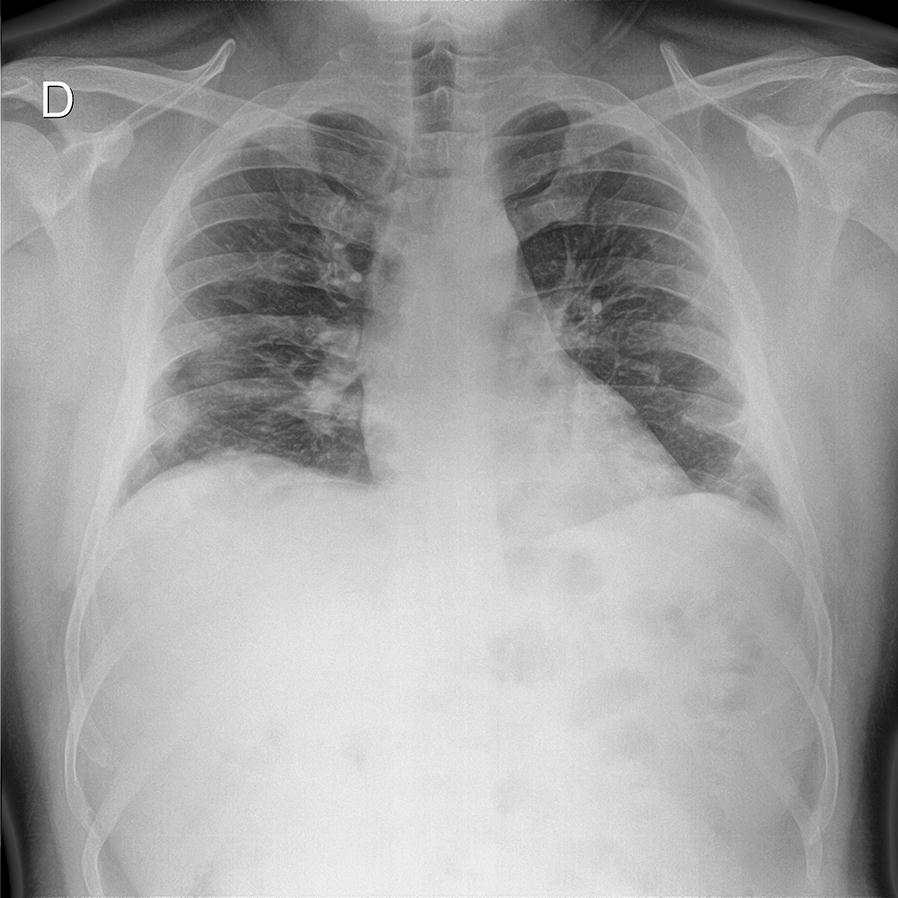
Chest radiograph obtained on admission shows peripheral ground-glass opacities in mid- and lower-third of the thorax
